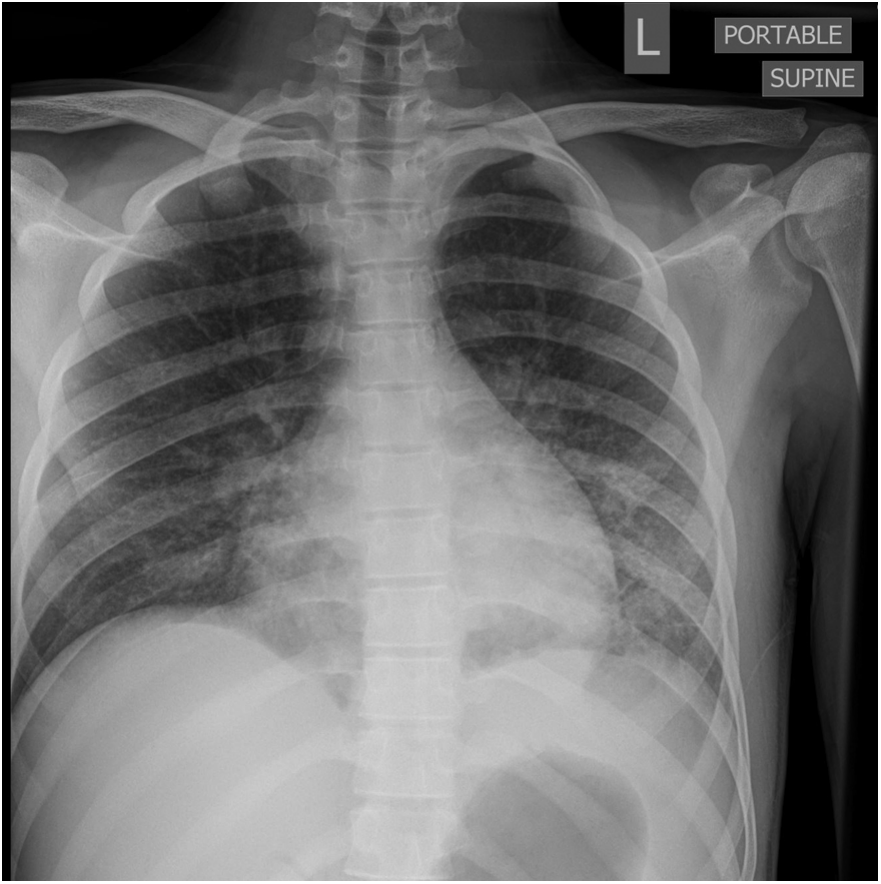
AP portable chest radiograph with diffuse but lower lobe predominant micronodular opacities and air bronchograms.
